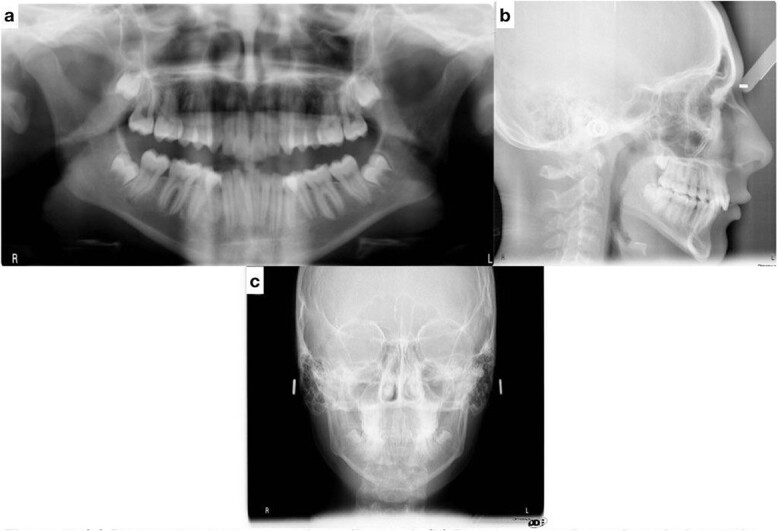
(a) Pretreatment panoramic radiograph; (b) pretreatment lateral cephalometric radiograph; (c) pretreatment posterior-anterior cephalometric radiograph.
